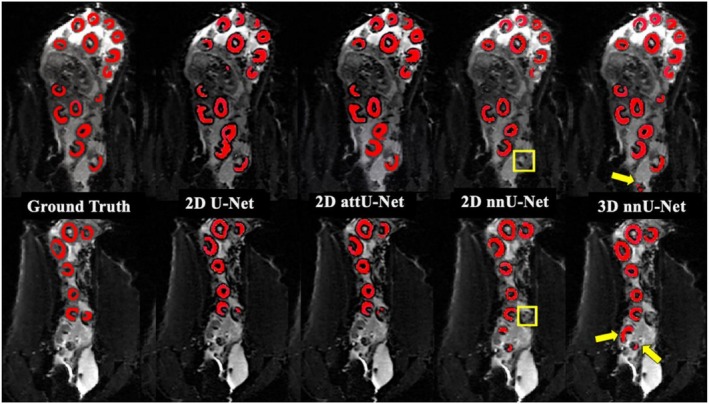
Qualitative comparison of placentome segmentation performance across models. Representative sagittal MRI slices are shown with red outlines denoting segmented placentomes. From left to right in each row: Manual gold standard contours, 2D U‐Net predictions, 2D attU‐Net predictions, 2D nnU‐Net predictions, and 3D nnU‐Net predictions. Yellow arrows highlight regions where the 3D nnU‐Net detects placentomes that were not detected in the gold standard (false positives), and yellow boxes indicate placentomes missed by the 2D U‐Net (false negatives).
